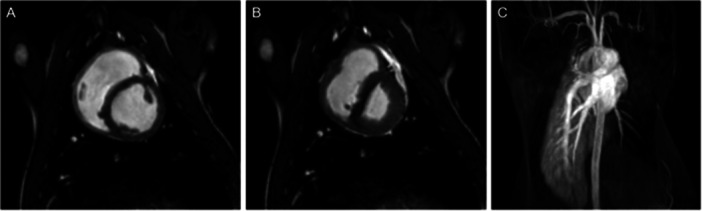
Functional cardiovascular MRI studies performed prior to sacrifice (Week 6) showing the dilatation of the right ventricle (A), flattening of the intraventricular septum towards the left ventricle (B) and complete perfusion defect of the left lung through the left pulmonary artery plug occlusion (C).
